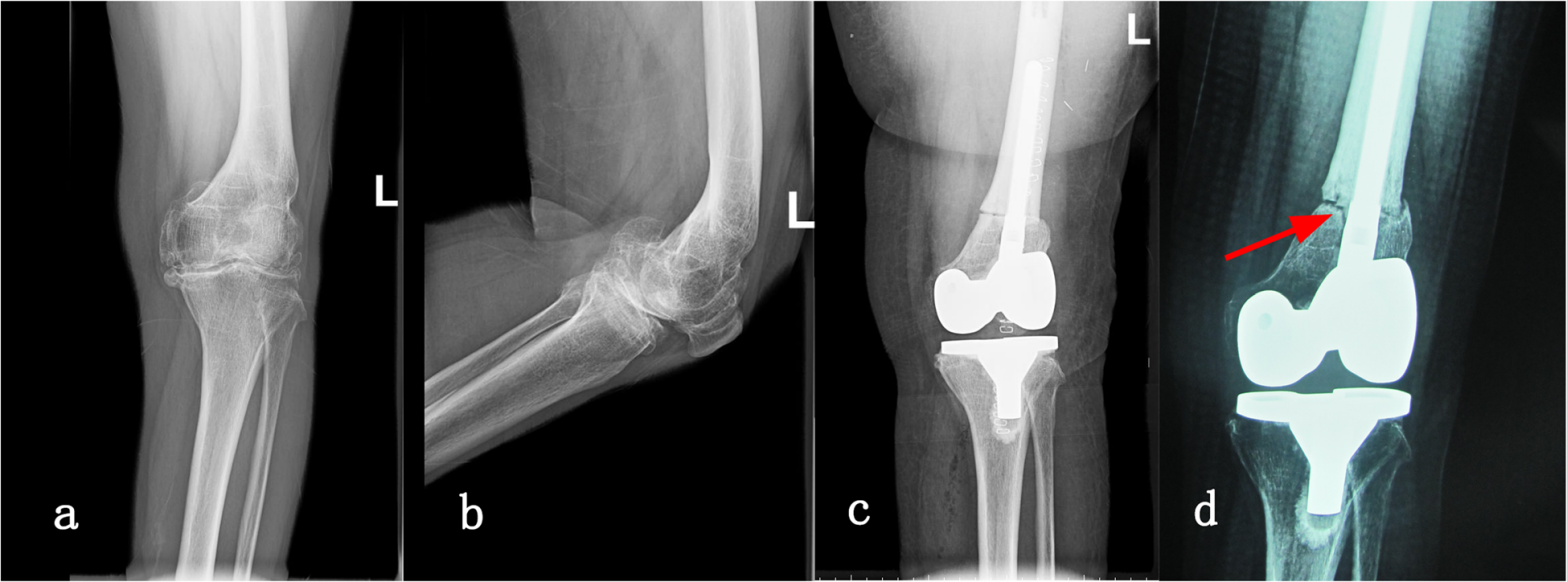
A 62-year-old women (case 3) with extra-articular deformity due to previous over-correction. a, b Anteroposterior view and lateral view before surgery. c X-ray at the first day after surgery. d Nonunion of the osteotomy resulting from the sandwiched cement
